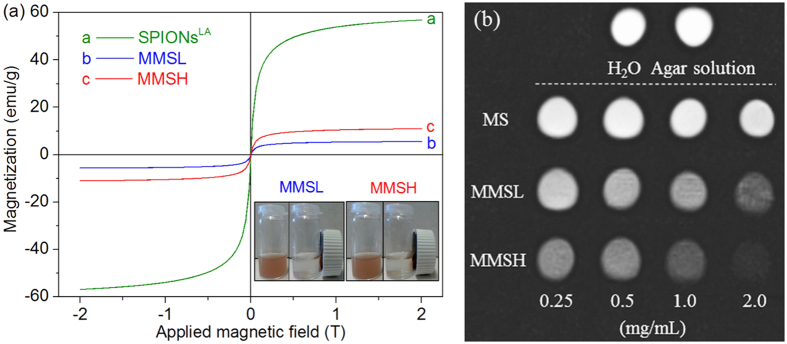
(a) magnetization curves measured at room temperature for SPIONs^LA^, MMSL and MMSH. The inset shows the response of MMSL and MMSH to a magnetic field. (b) *T*_2_-weighted MR images of H_2_O, 0.1 wt% agar solution and 0.25–2.0 mg/mL of MS, MMSL and MMSH dispersed in 0.1 wt% agar solution.
